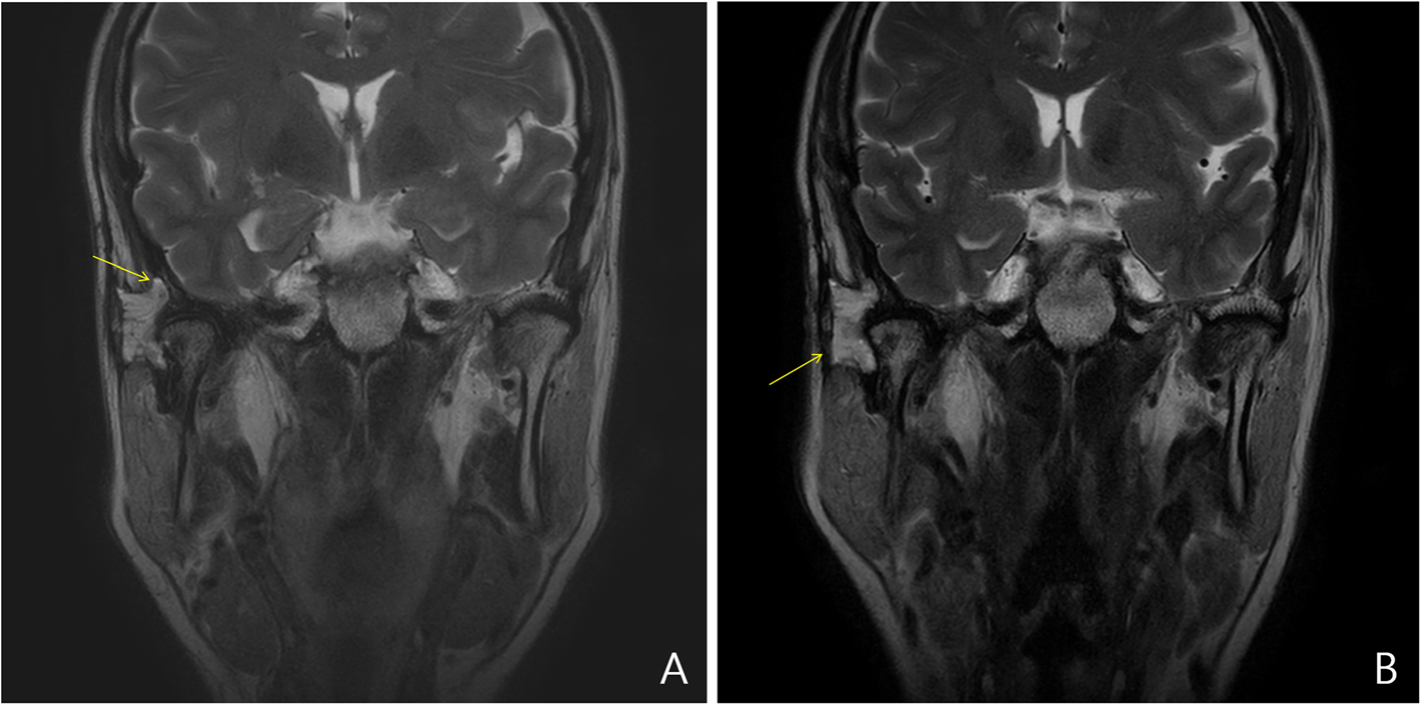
Magnetic resonance imaging (A) coronal scan, 7 months postoperatively, shows the stable inguinal fat pad graft covering the temporal bone defect (arrow) and do not indicate chondroblastoma recurrence. At the 18-month follow-up visit, the magnetic resonance imaging (B) coronal view fails to reveal newly developed, abnormally enhanced lesions or restricted diffusion into the operation bed (arrow). There is no evidence of local recurrence
